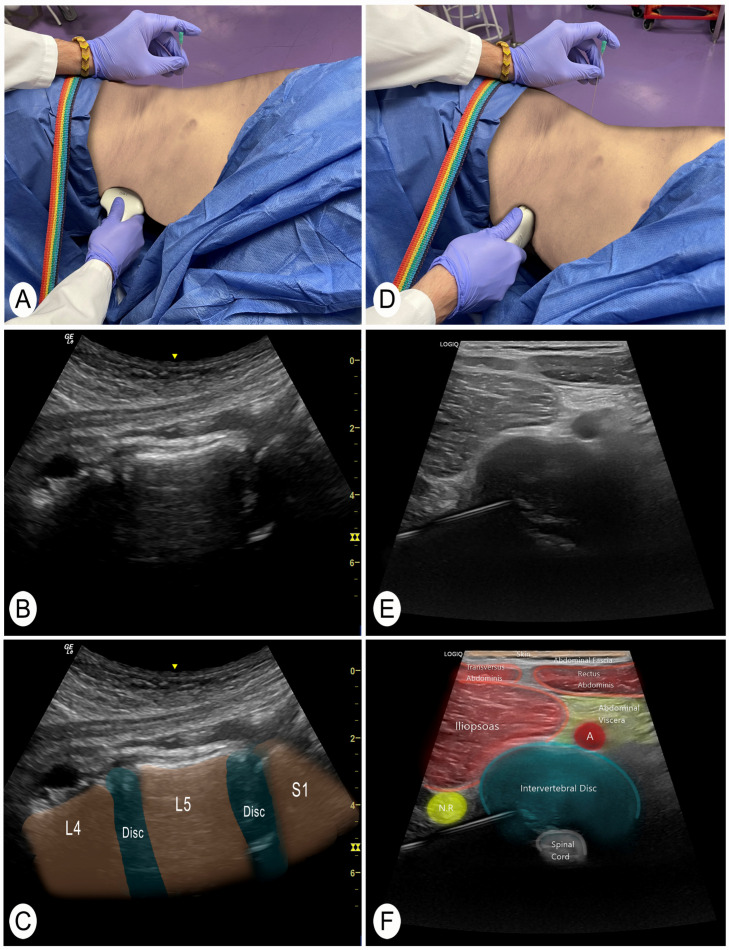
Anterior and slightly lateral view of the intervertebral disc. Abbreviations: N.R., nerve root; A, artery. L4, 4th lumbar vertebra; L5, 5th lumbar vertebra; S1, 1st sacral vertebra. (A) Position to locate the vertebral level with the transducer in a longitudinal slice of the vertebrae from the abdomen. (B) Ultrasound image of the longitudinal section of the lumbar levels from a ventral view. (C) Ultrasound image of the longitudinal section of the lumbar levels from a ventral view with the vertebral segments identified. (D) Position of the transducer in a cross-section of the intervertebral disc previously punctured. (E) Cross-sectional ultrasound image of the intervertebral disc from a ventral view where the previously inserted needle is located and adjusted. (F) Ultrasound cross-sectional identification of the structures.
